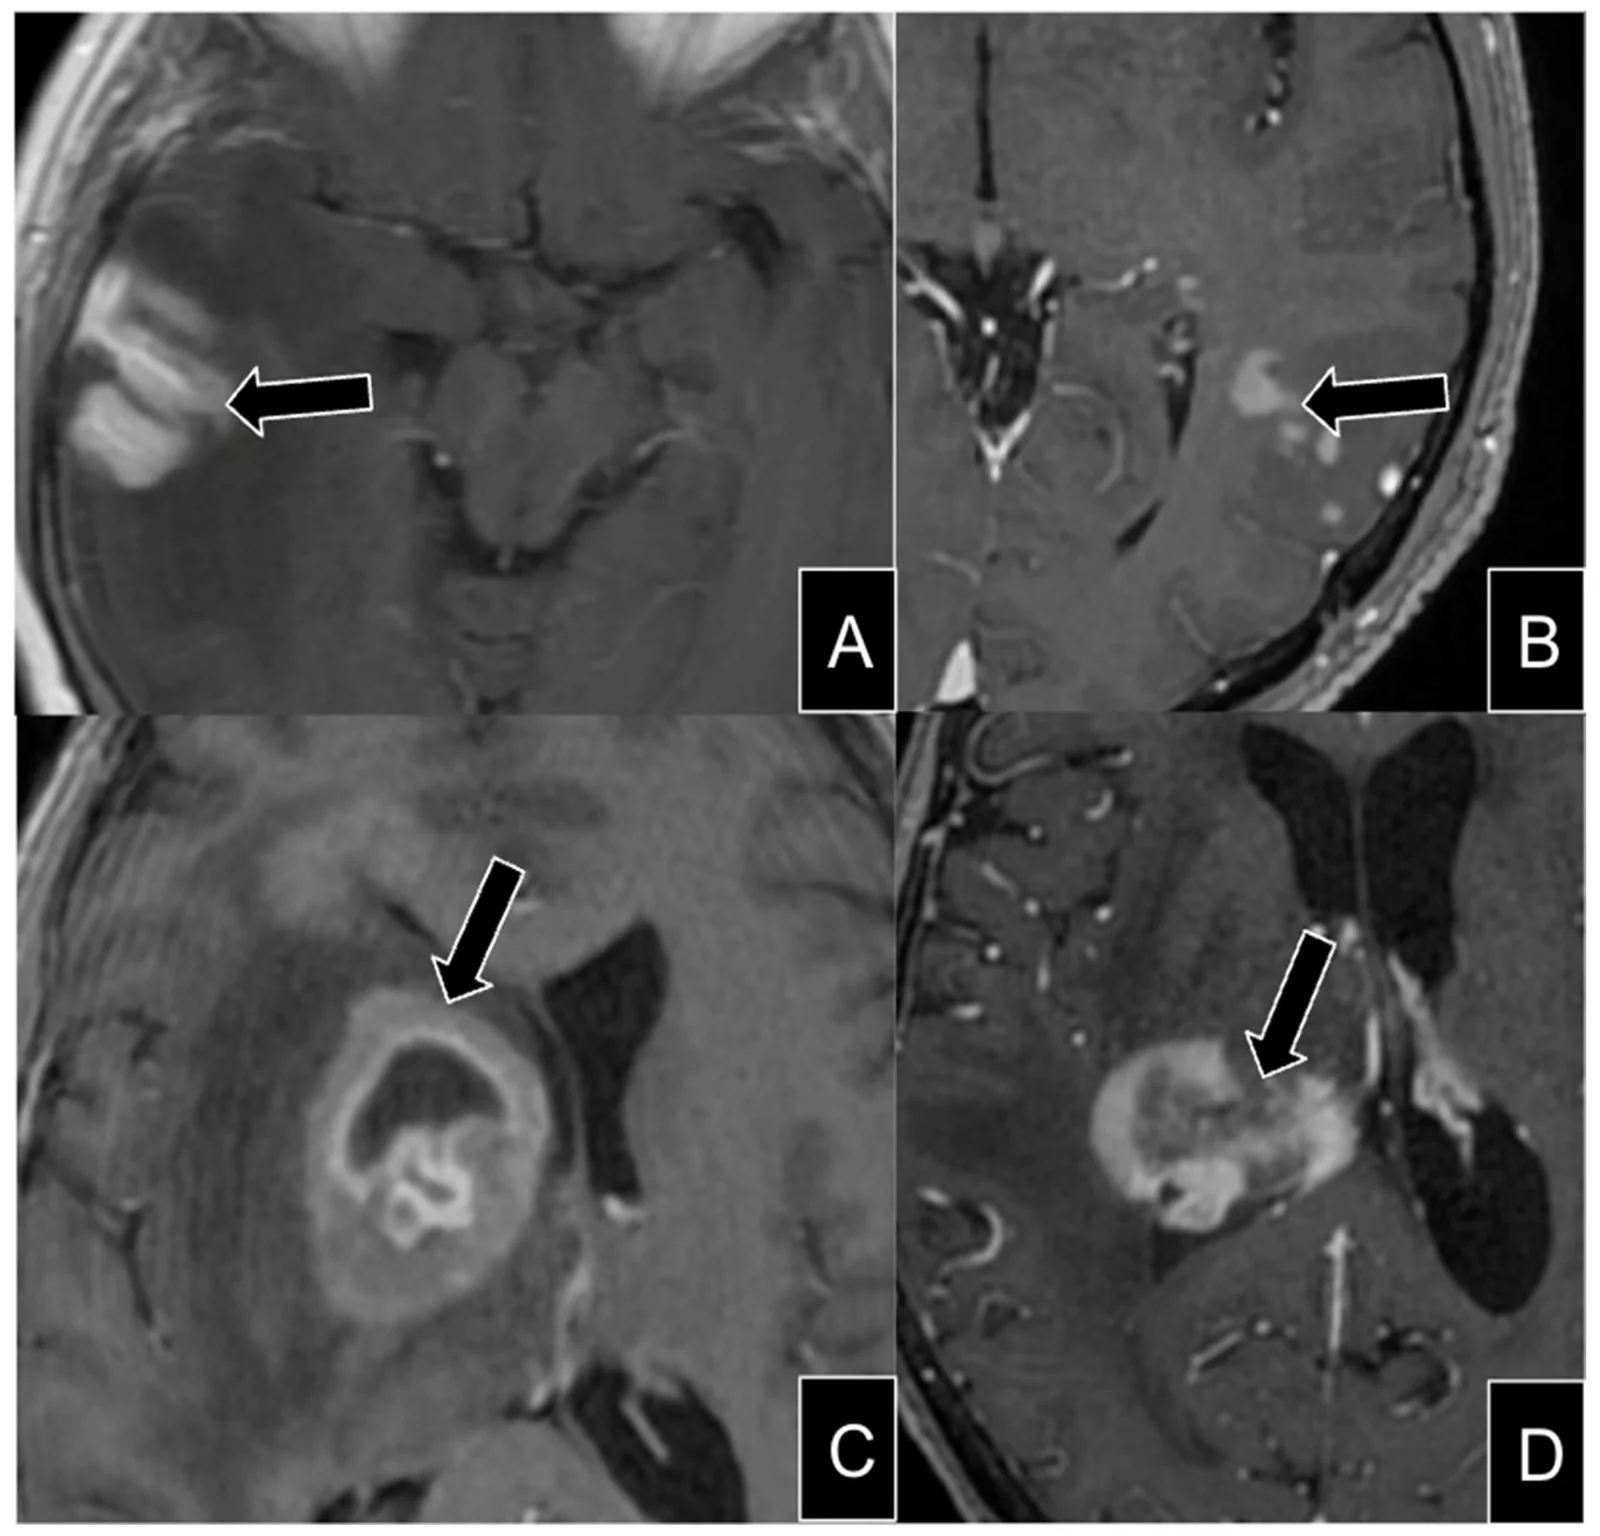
Enhancement pattern of large B-cell lymphoma of immune-privileged sites. Axial MRI showing variable enhancement patterns (black arrows), including gyriform (A), punctiform (B), nodular with cystic component (C), irregular and heterogeneous (D).
